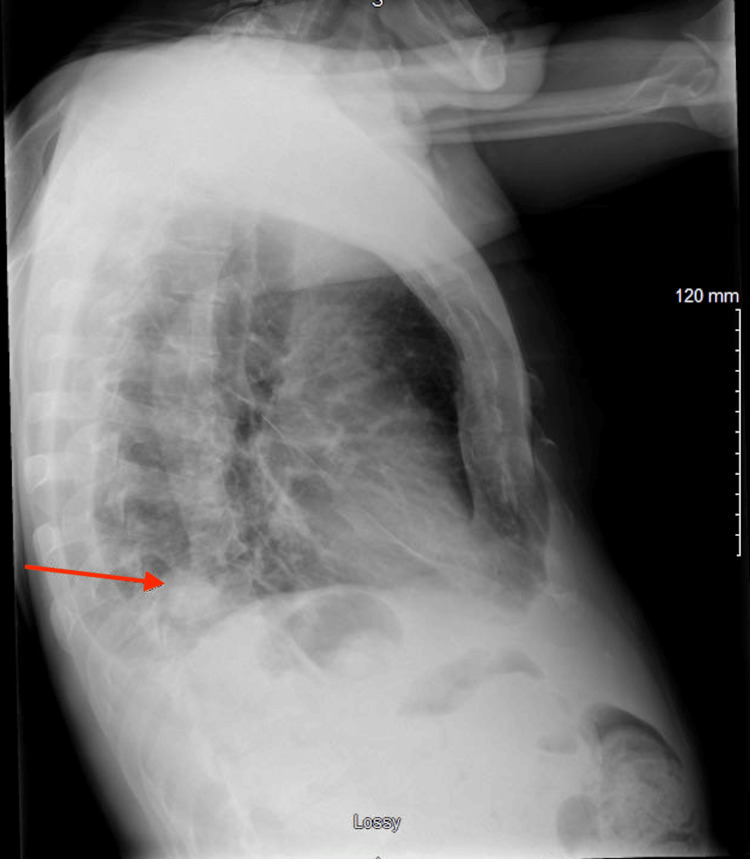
Lateral chest X-ray depicting opacity in the right lower lobe.
